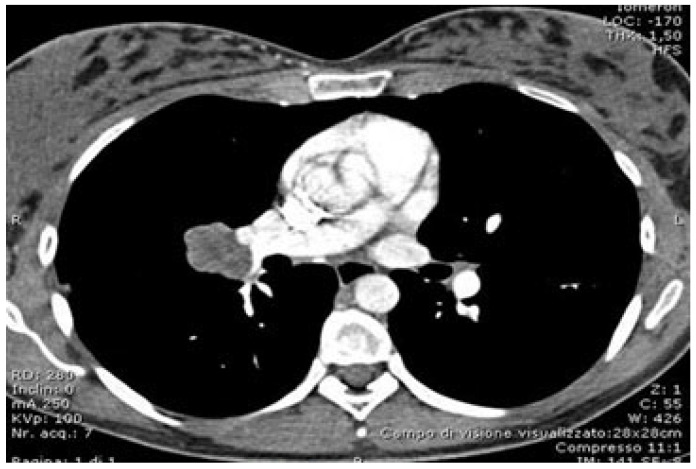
CT scan showing the target lesion.
